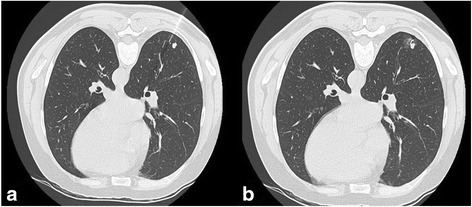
A 66-year-old patient with a solitary pulmonary nodule of unknown origin and situated in the right lower lobe, thus scheduled for VATS. (a) After CT-acquisition in prone position the 20-gauge needle is introduced (b), followed by the deployment of the hook wire, accidently causing a focal, small and asymptomatic pneumothorax
